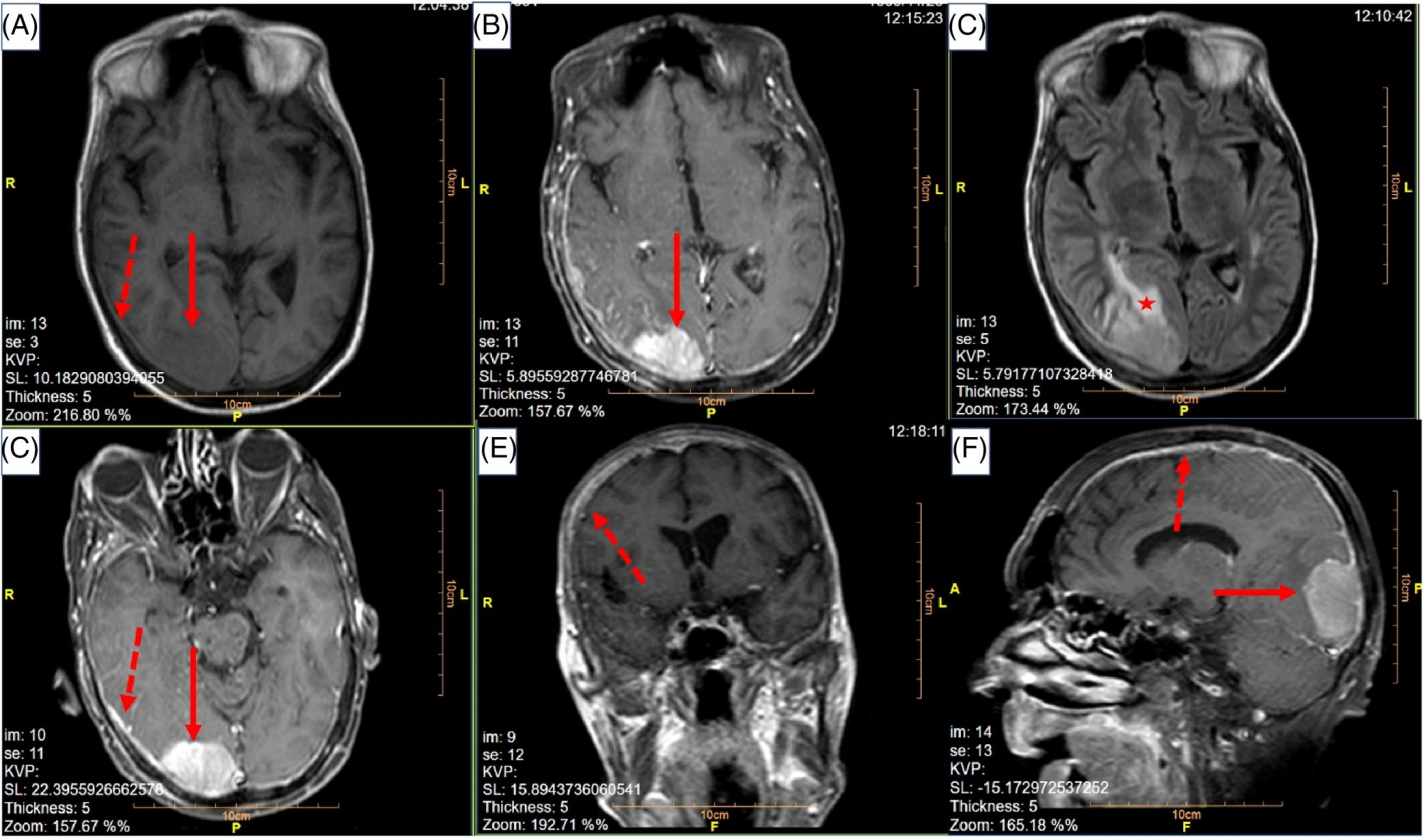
MRI brain obtained from (A) axial plane, T1 without gadolinium contrast, (B) axial plane, T1 with contrast, (C) axial plane, T2, (D) axial plane, T1 with contrast, (E) coronal plane, T1 with contrast, and (F) sagittal plane, T1 with contrast. Solid lines show the 50 × 40 mm interaxial mass at right occipital lobe which was hypo/isosignal at T1‐weighted images and hypersignal at T2‐weighted images, with enhancement of the lesion at contrast‐enhanced T1‐weighted images. Dashed lines show diffuse leptomeningeal enhancement. The star shows the perilesional vasogenic edema
